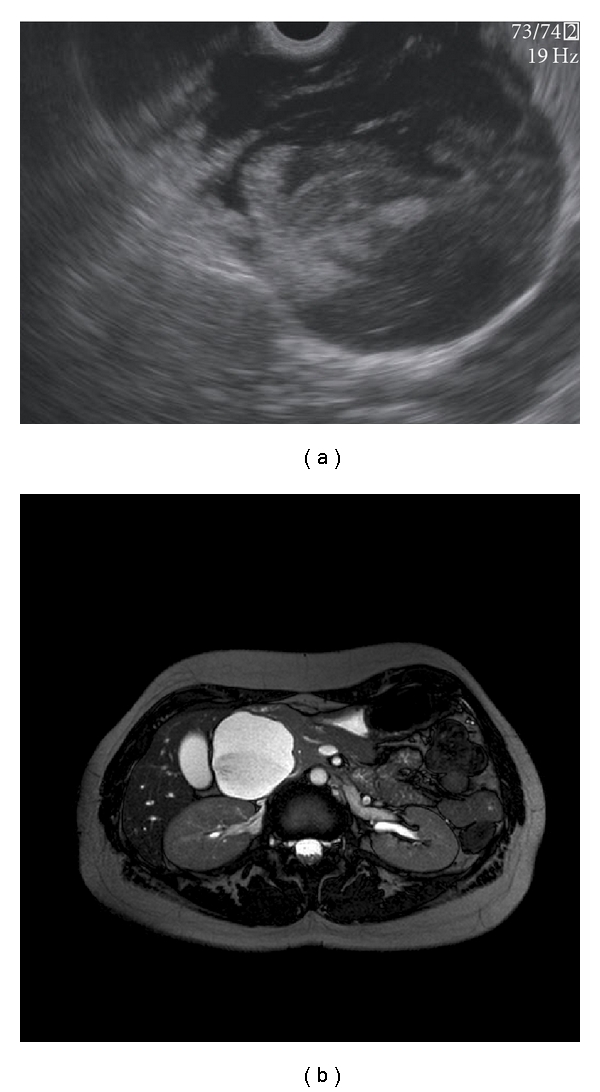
(a) EUS image of a malignant IPMN in the head of the pancreas. (b) MRI image of a malignant IPMN in the head of the pancreas.
